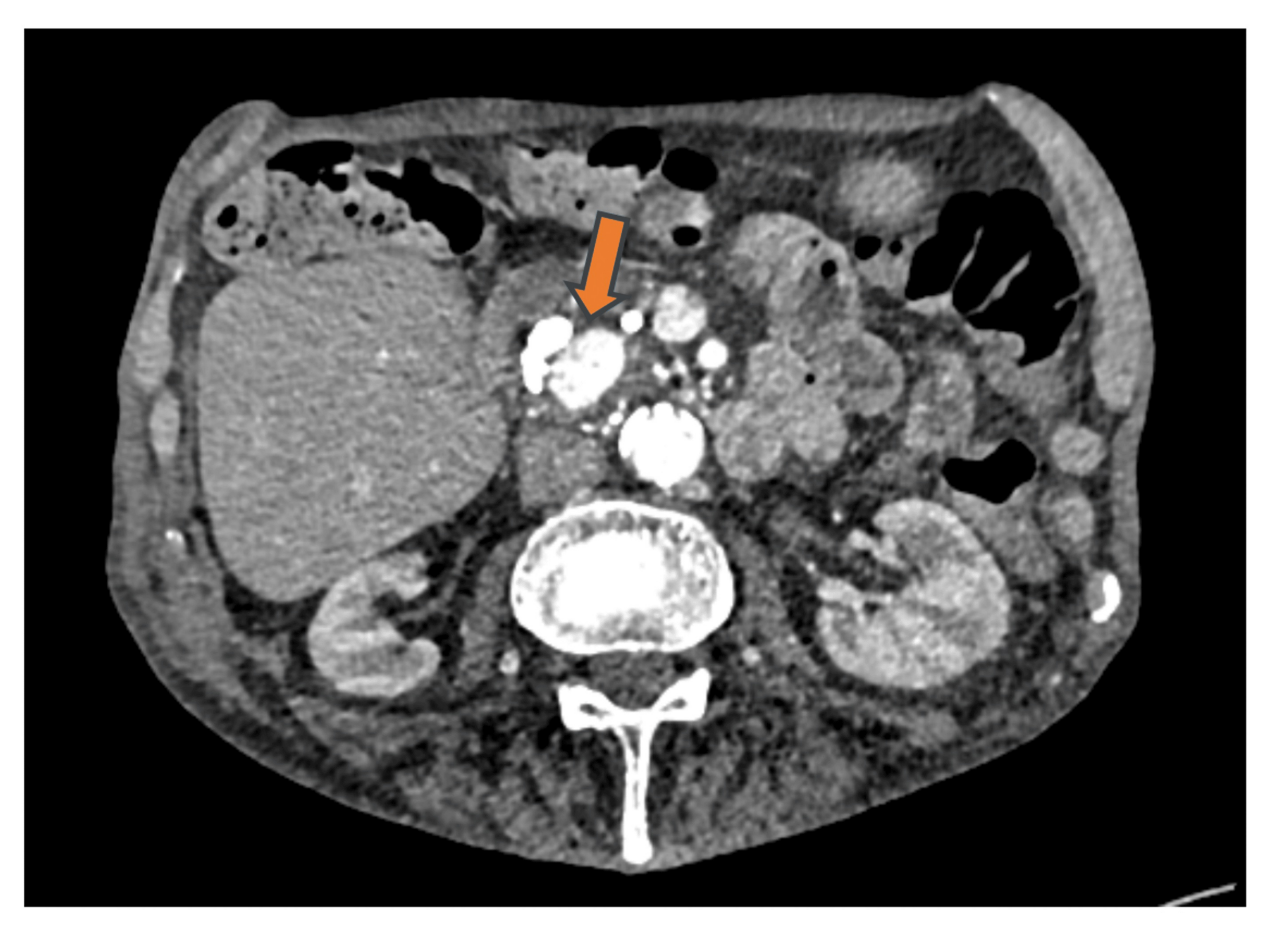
Contrast-enhanced CT scan of the abdomen showing a 3.3 cm × 1.4 cm × 2.2 cm aneurysm involving the head and uncinate process of the pancreas.
